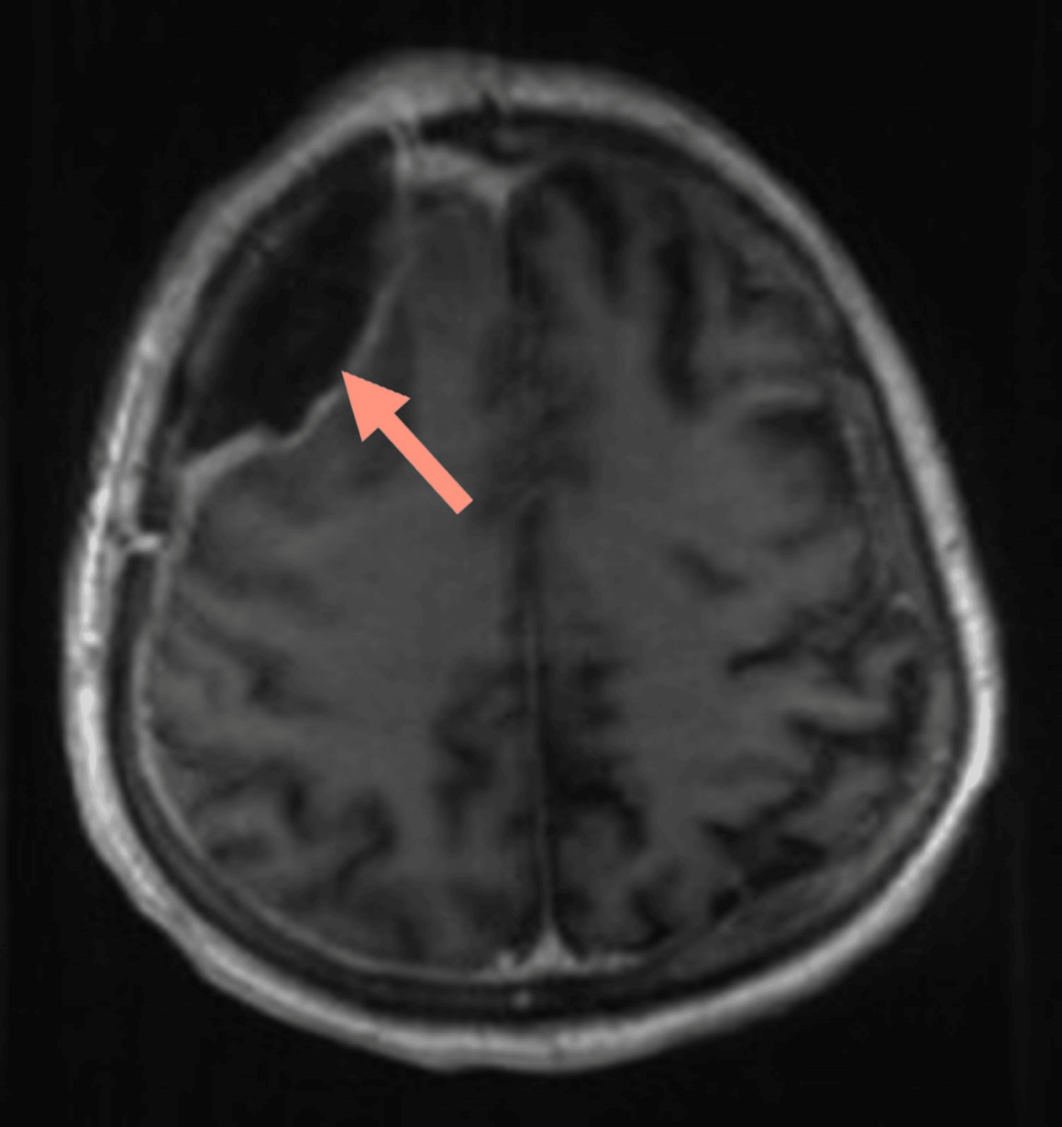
Axial T1-weighted MRI post contrast shows dural enhancement of the intracranial extra-axial extension of the tumor (red arrow).
